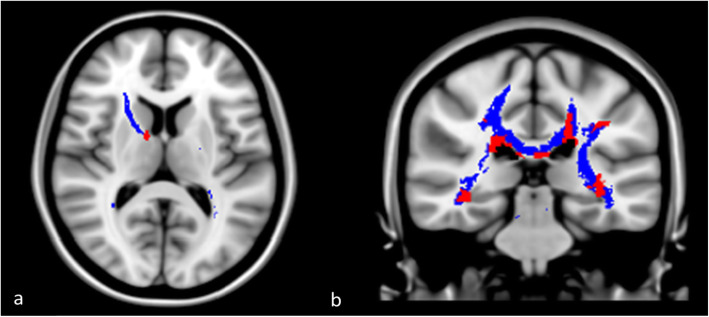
Lesion and disconnectome maps. T1-weighted hypointense lesion (*red*) and disconnectome (*blue*) maps obtained in two patients involved in the study are shown on the Montreal Neurological Institute 152 template. Axial plane (a): a little lesion in the genu of internal capsule (*red*) leads to a more extensive white matter bundle disconnection involving the entire anterior arm of internal capsule (*blue*). Coronal plane (b): multiple lesions in periventricular areas (*red*) lead to a very extensive white matter bundle disconnection (*blue*), especially in the corpus callosum
